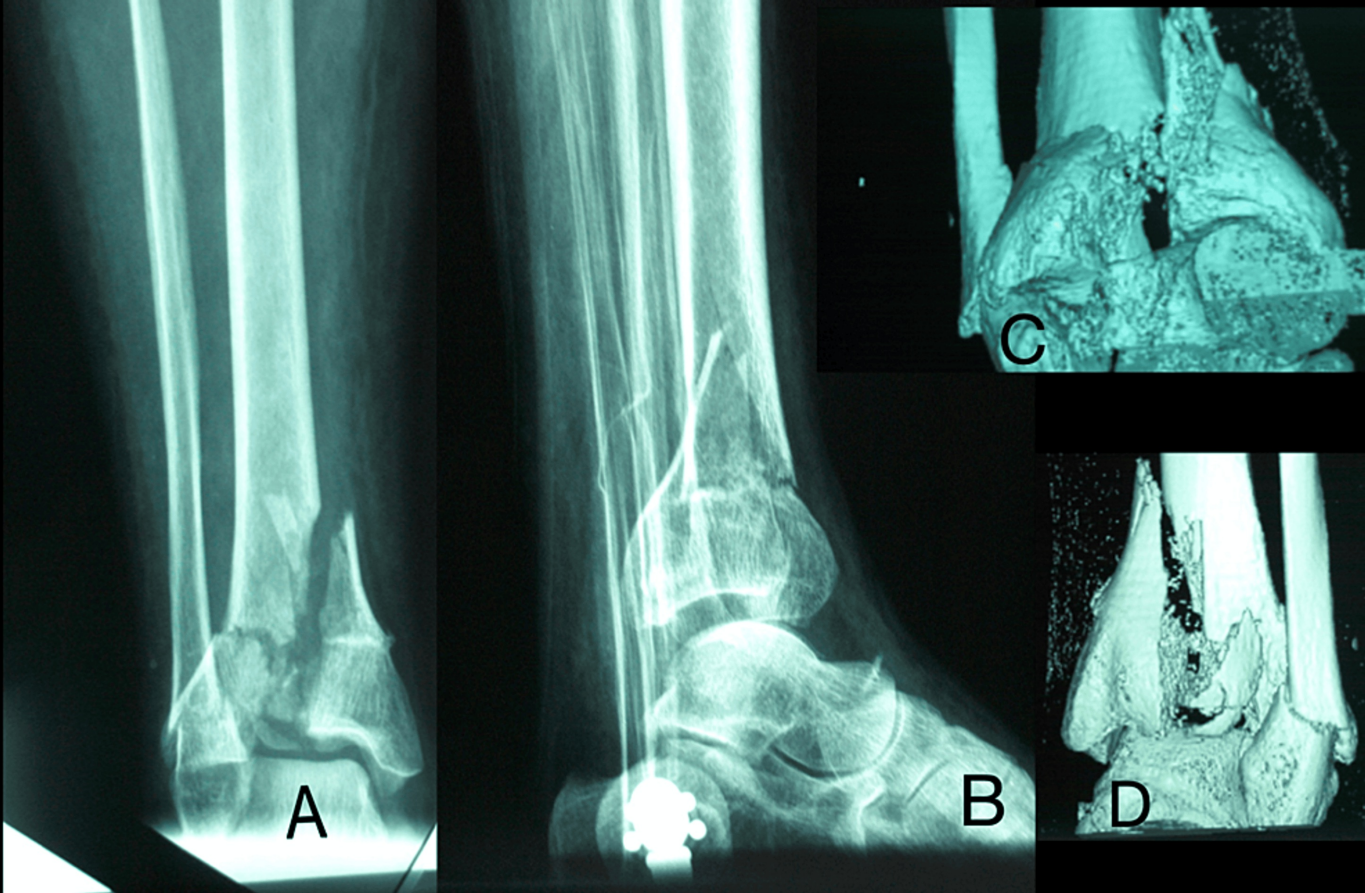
Anteroposterior (A), lateral (B) radiograph and 3D reconstruction CT scan (C,D) demonstrating the explosive nature of the severe pilon injury.
